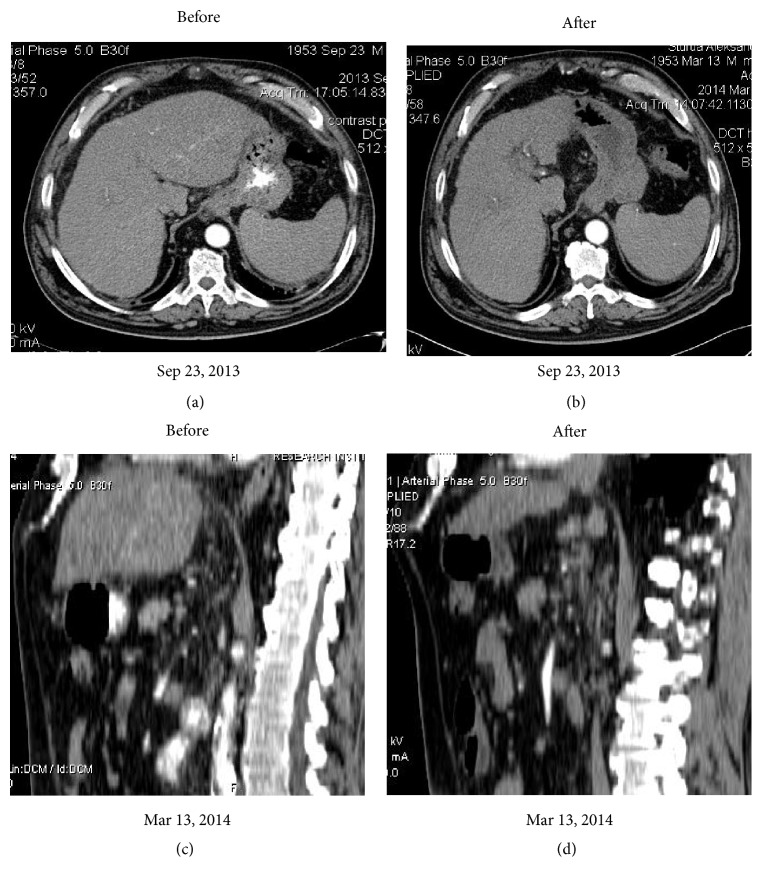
Comparison: CT before and in 5 months after VesOpen procedure. Note the decreased size and normal shape of the left lobe.
